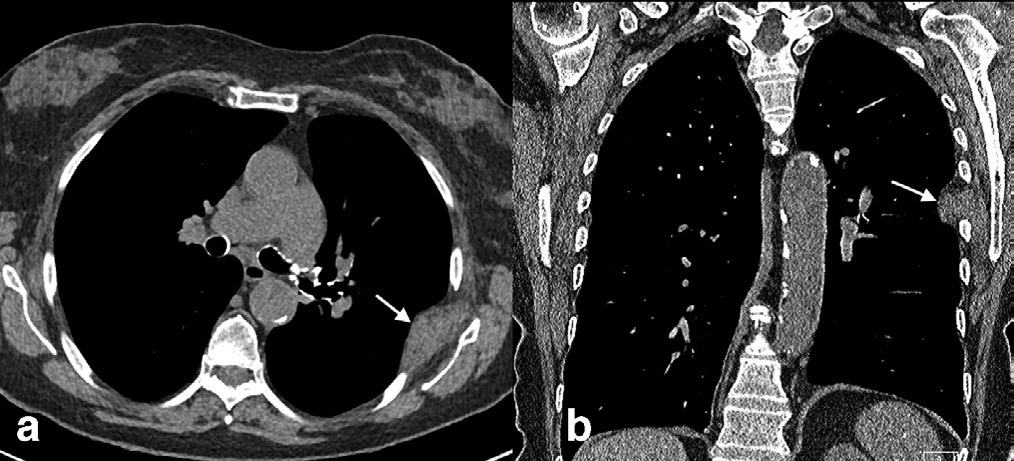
(a, b) Axial and coronal CT images in an 80-year-old female (Case 2) showing a unilateral left-sided mixed soft tissue and fatty mass herniating medially through a widened rib interspace at a prior thoracotomy site.
